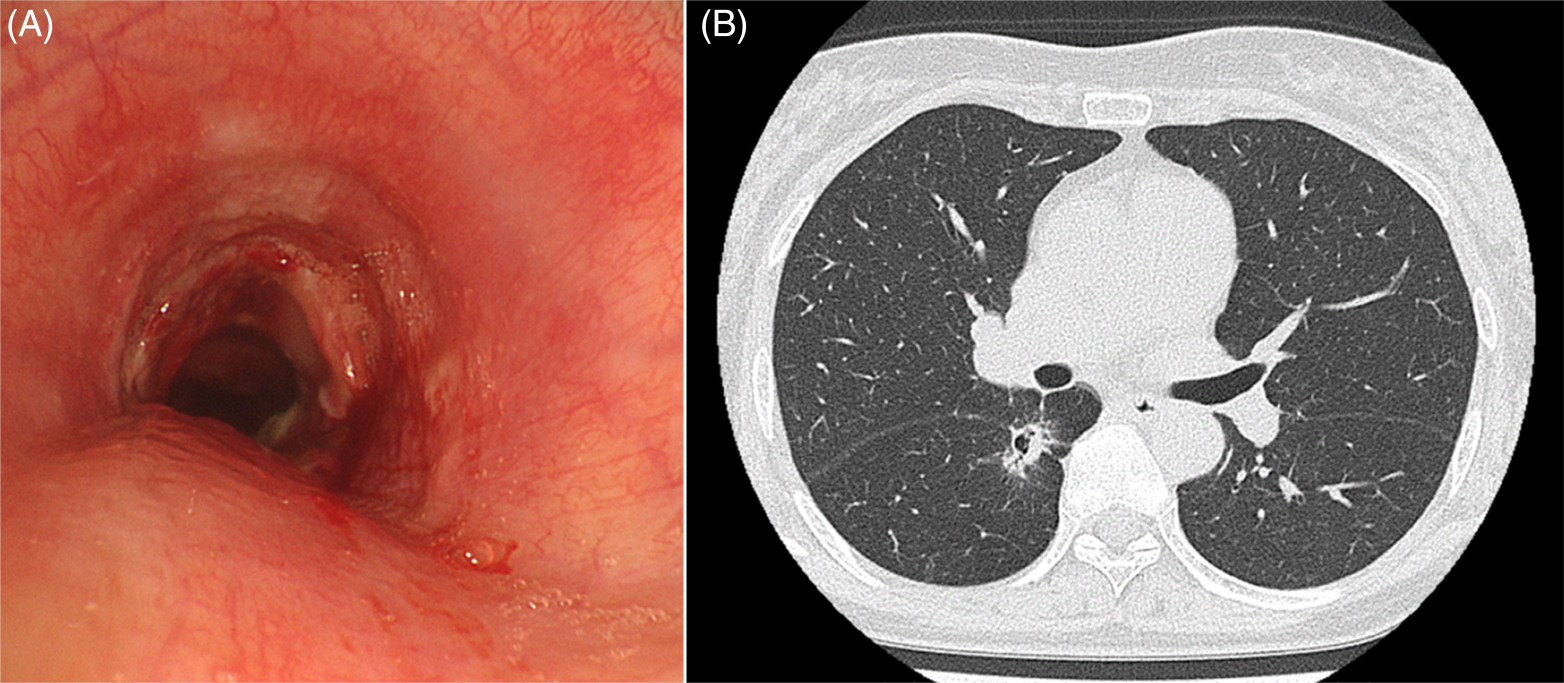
(A) Bronchoscopic image obtained after AERO stent removal. (B) Chest CT shows a mass in the S6 segment of the right lower lobe successfully treated with osimertinib.
